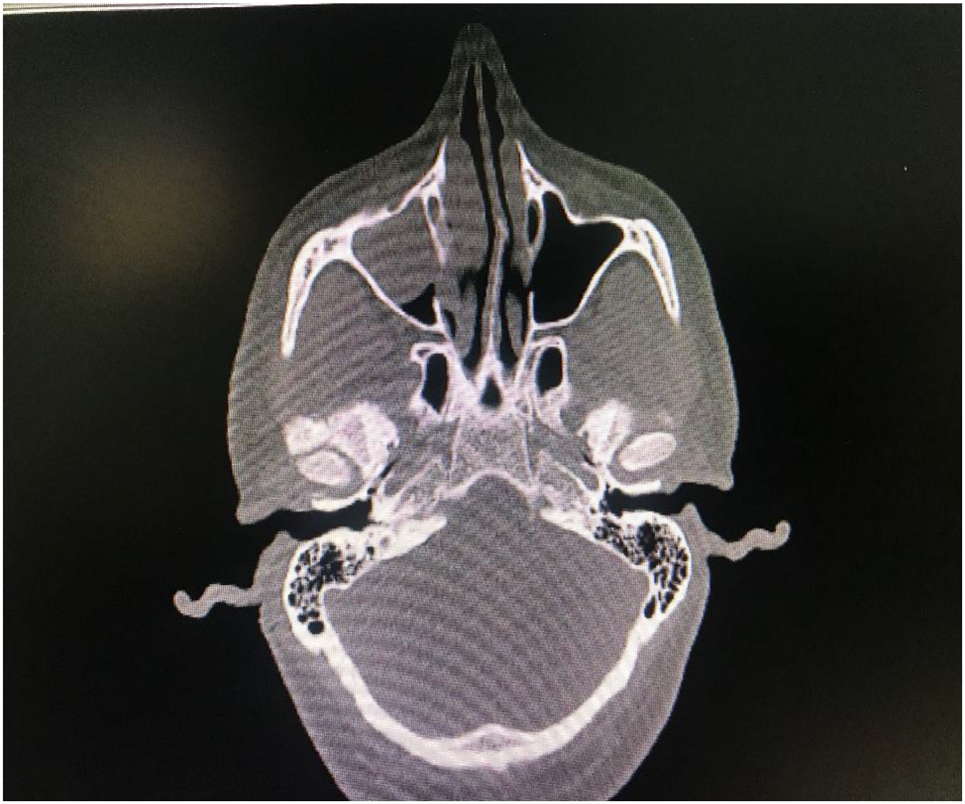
Axial CT head showing: almost total opacification of the right maxillary sinus with concentric soft tissues mass protruding into the nasal cavity deviating the nasal septum.
